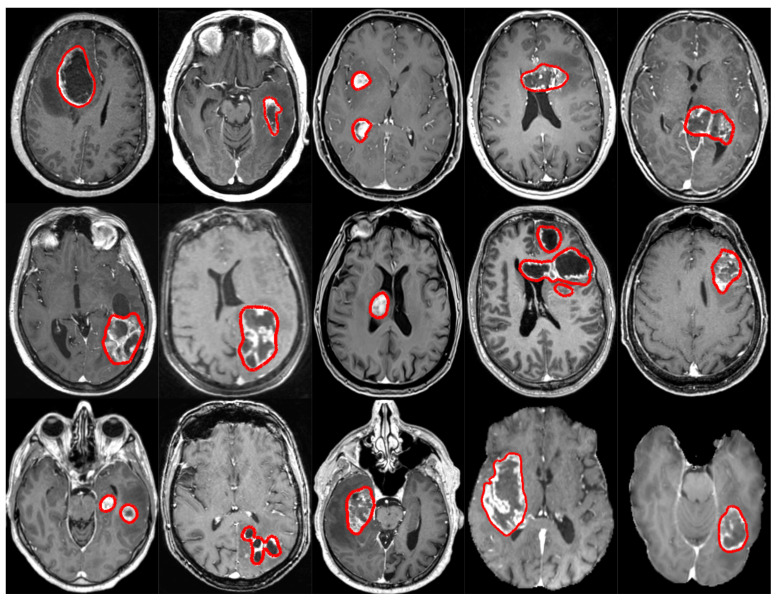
Example axial views of T1-weighted MRI volumes used in the study, with manual segmentations outlined in red. One case from each hospital is shown, and the rightmost two cases featured in the last row are from the BraTS challenge dataset.
